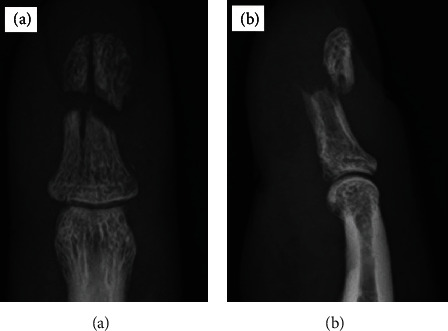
Radiographs showing nonunion of the distal phalanx of the right middle finger 10 weeks after injury: (a) AP view; (a) lateral view.
